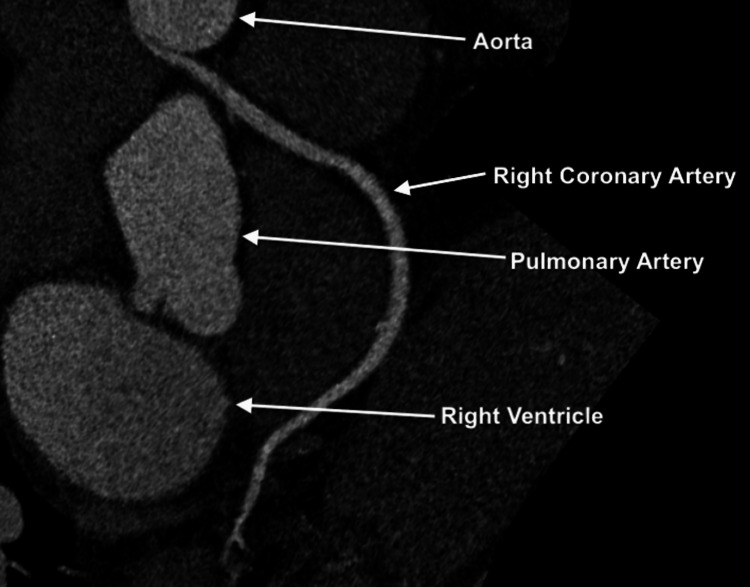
CTA image showing the anomalous origin of the RCA in this patient, as well as the interarterial course. CTA image showing the interarterial course of the RCA. The anomalous RCA is seen, arising from the left sinus of Valsalva and coursing between the aorta and the PA. Note the oblique origin and the intramural course within the aortic wall, all factors compromising coronary blood flow. CTA: computed tomography angiography; RCA: right coronary artery; PA: pulmonary artery
